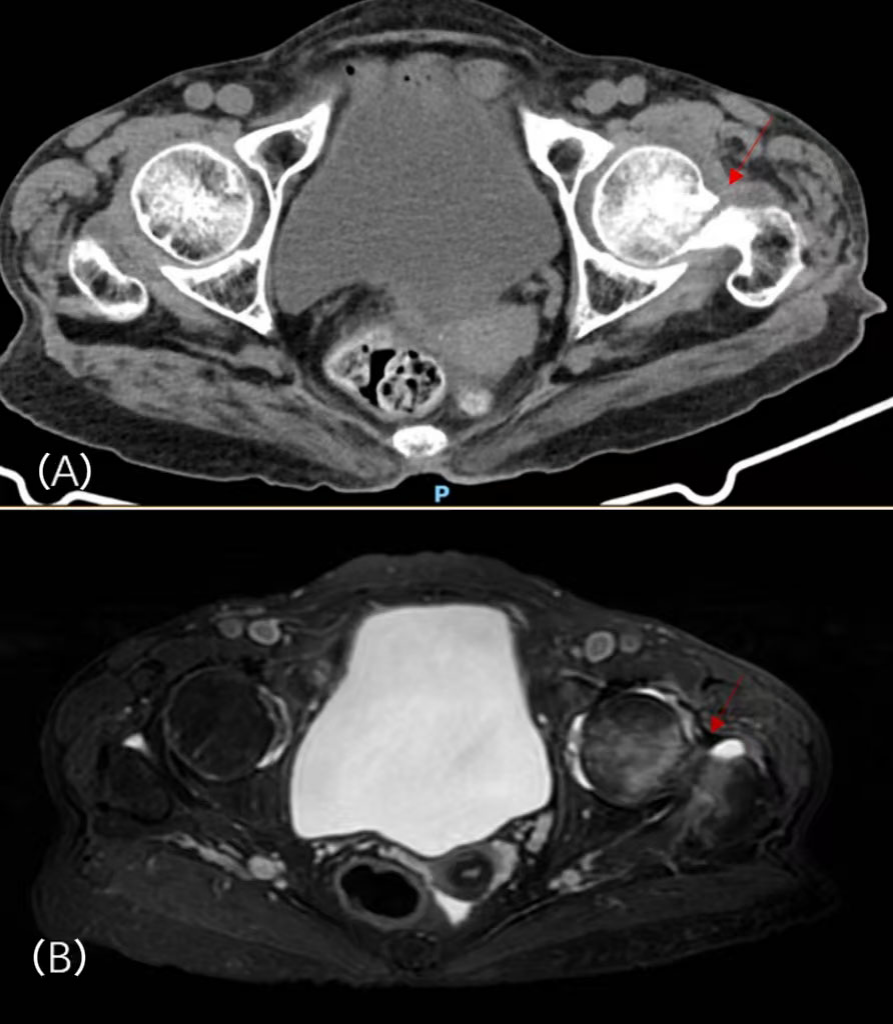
Hip CT (A) and hip MRI (B) before treatment.
